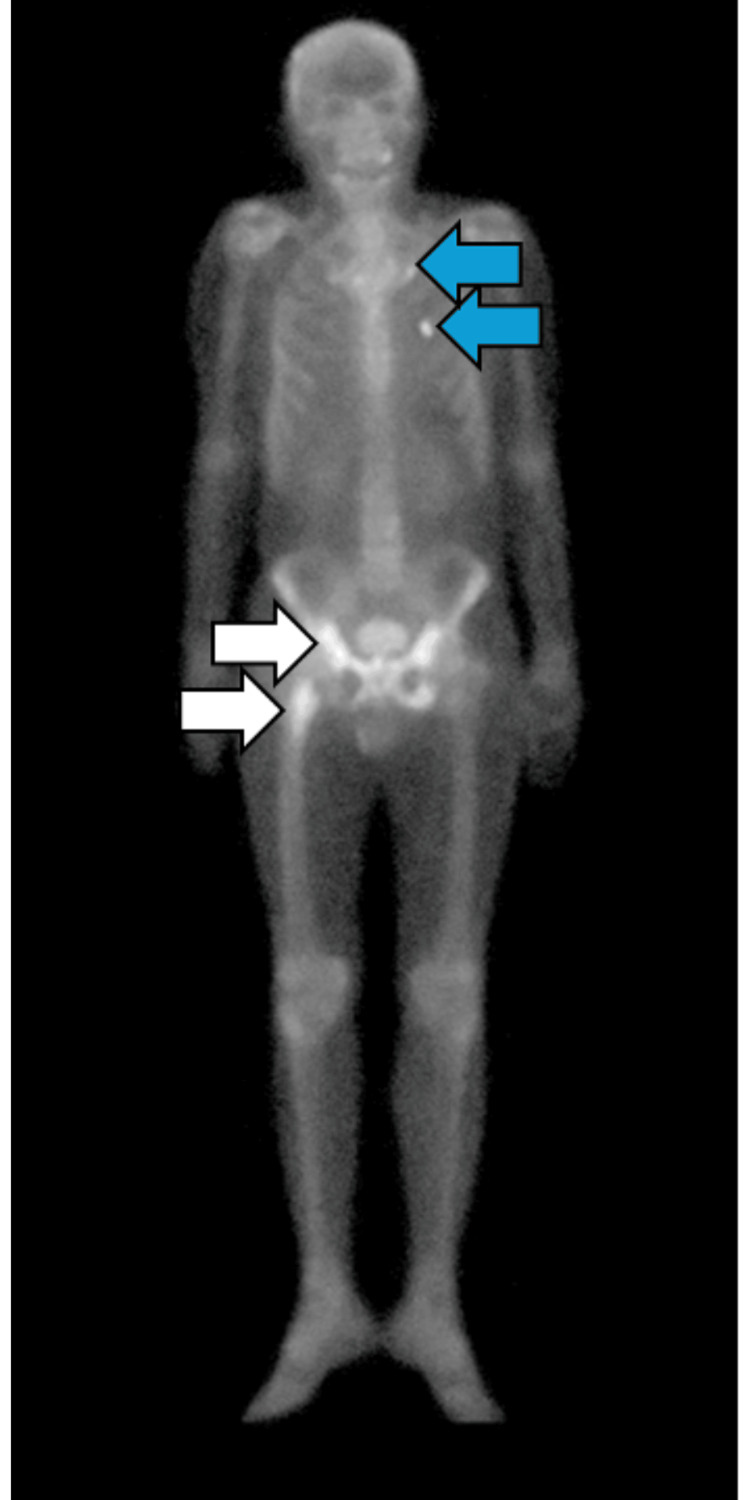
Bone scintigraphy demonstrating osseous metastases. White arrows show increased radiotracer uptake in the right pelvis and proximal right femur, while blue arrows show calcifications at the costochondral junctions of the left first and third ribs.
